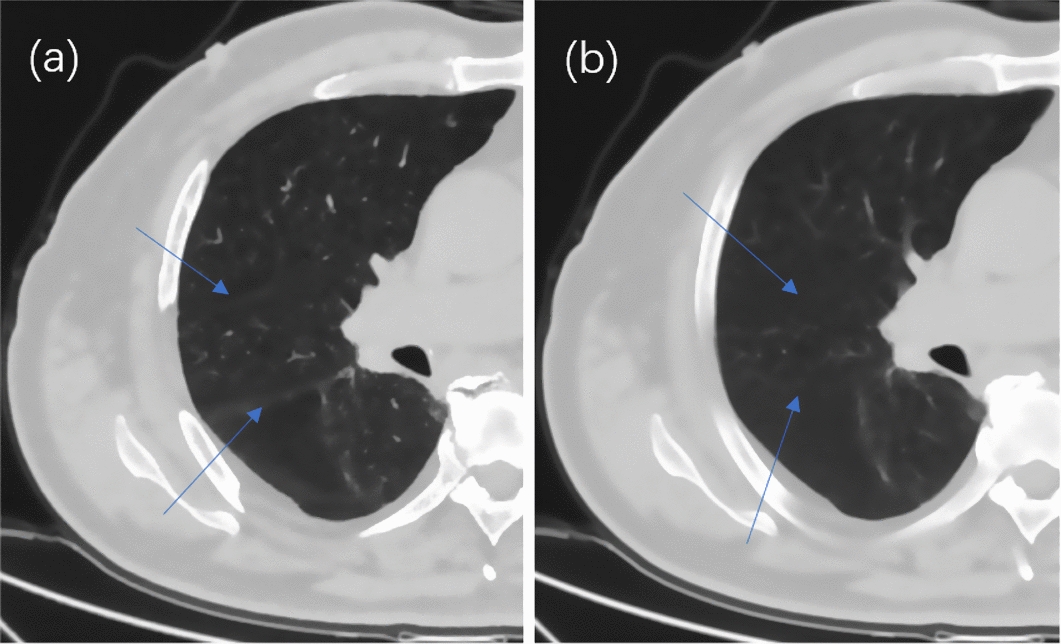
Axial view of right lung for one patient. Blue arrows point to the fissures in right lung. a 1 mm slice CT, b 5 mm slice CT
